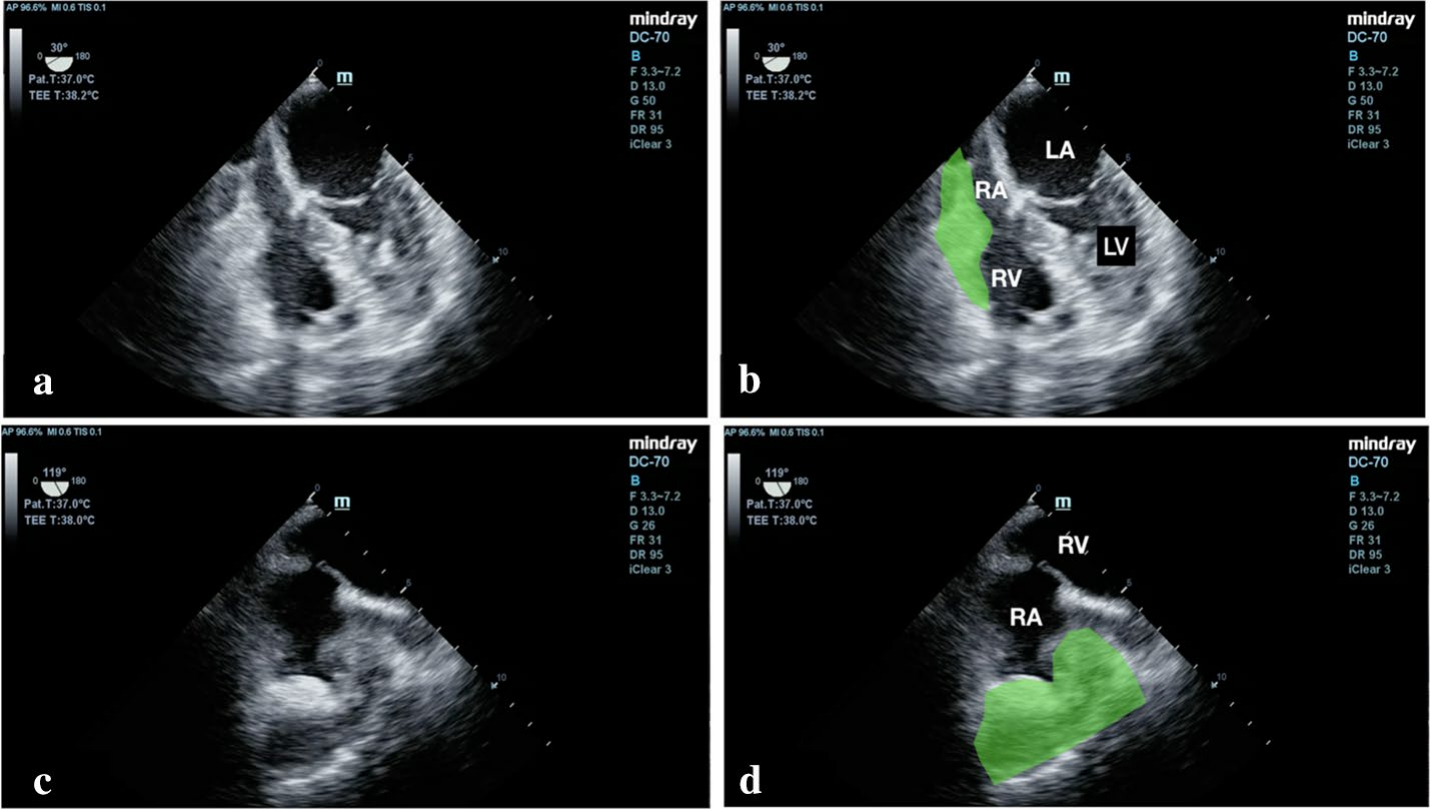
a, b Transesophageal echocardiogram (TEE) mid-esophageal 4-chamber view showed a compressed right atrium and posterior pericardial clot (green watermark). c, d TEE bicaval view showed similar findings with right atrium compression by an extrinsic posterior pericardial clot (green watermark). *LA* left atrium, *LV* left ventricle, *RA* right atrium, *RV* right ventricle
